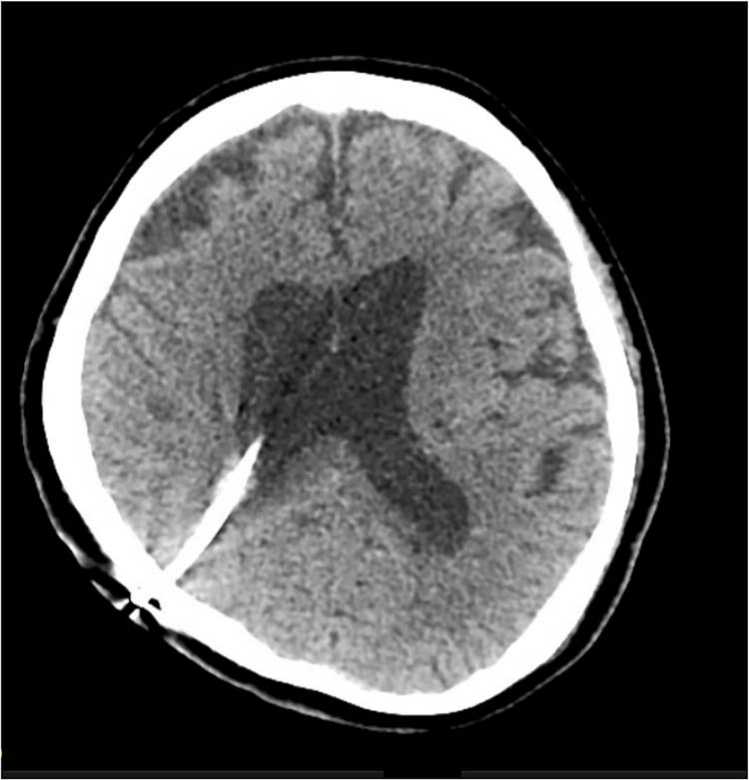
CT scan performed 41 days after shunt revision, showing resolution of the periventricular cyst.
